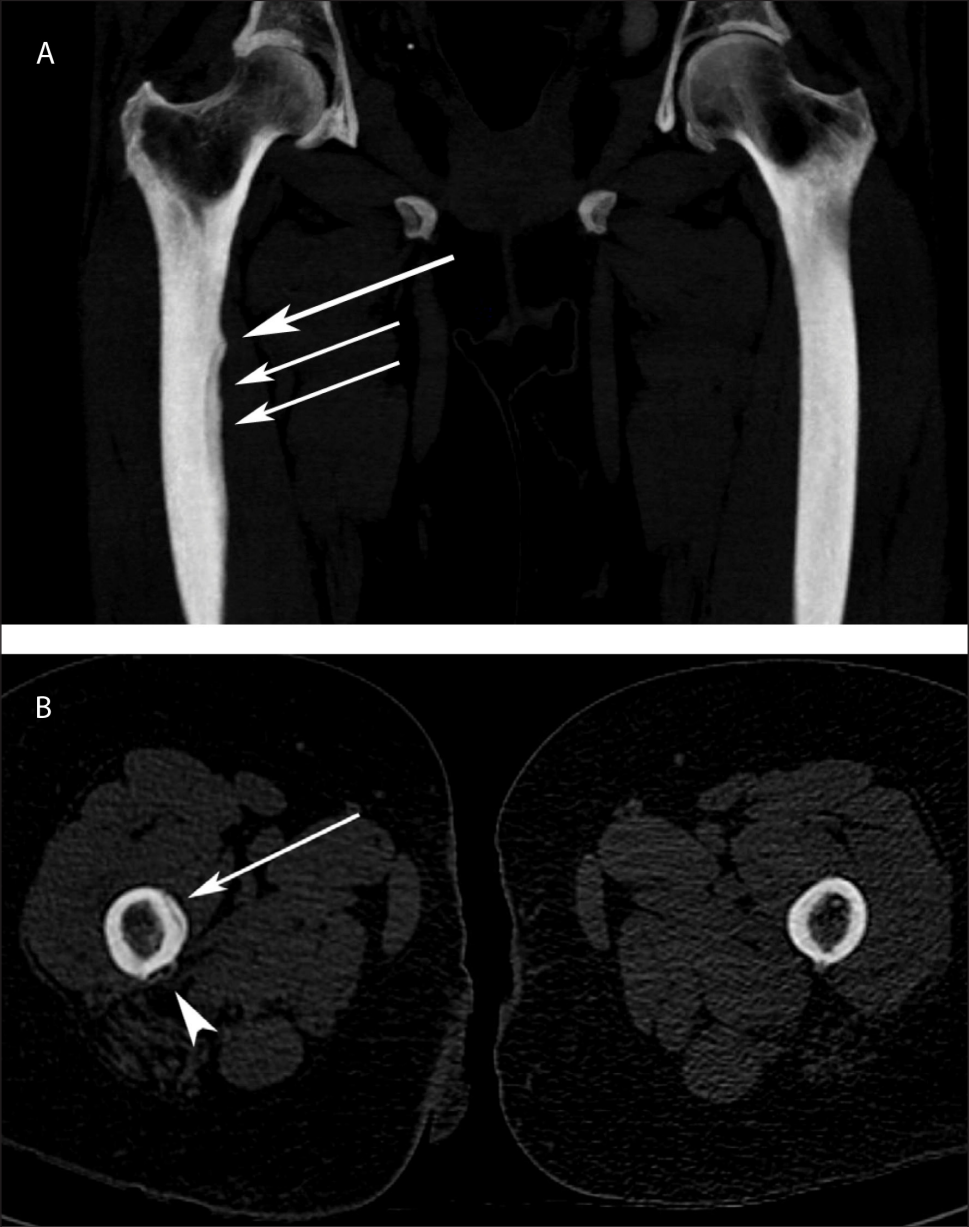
a) Coronal CT maximum intensity projection reconstruction of the proximal femur shows medial periosteal thickening and fine line of lucency consistent with stress fracture (arrows). b) Axial CT image of the proximal femur shows the medial stress fracture (arrow) with a periosteal reaction on its posteromedial part (site of adductor’s avulsion) (arrowhead).
